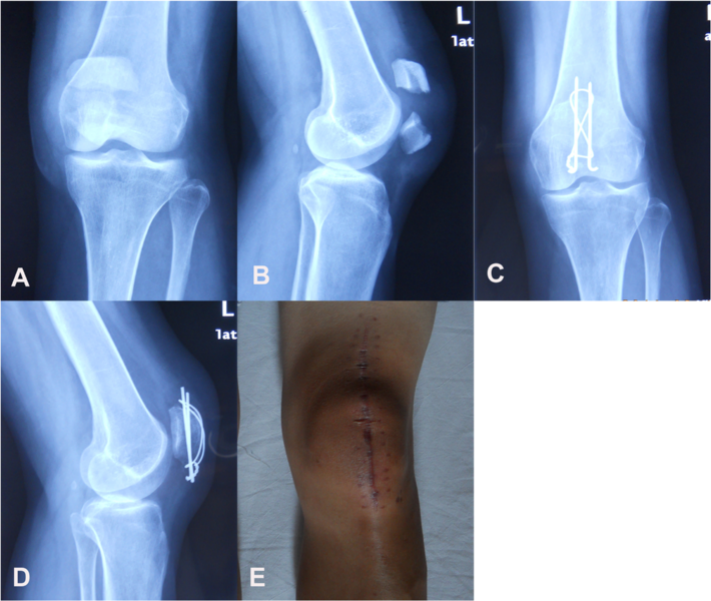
Open reduction and fixation using a modified tension band. a and b, Preoperative radiograph of a 48-year-old woman who had a transverse patella fracture. c and d, Postoperative radiograph showing the proper placement of wire and Kirschner wire. e The knee joint anterior midline approach showing that a long skin incision of approximately 14 cm was required in this case
